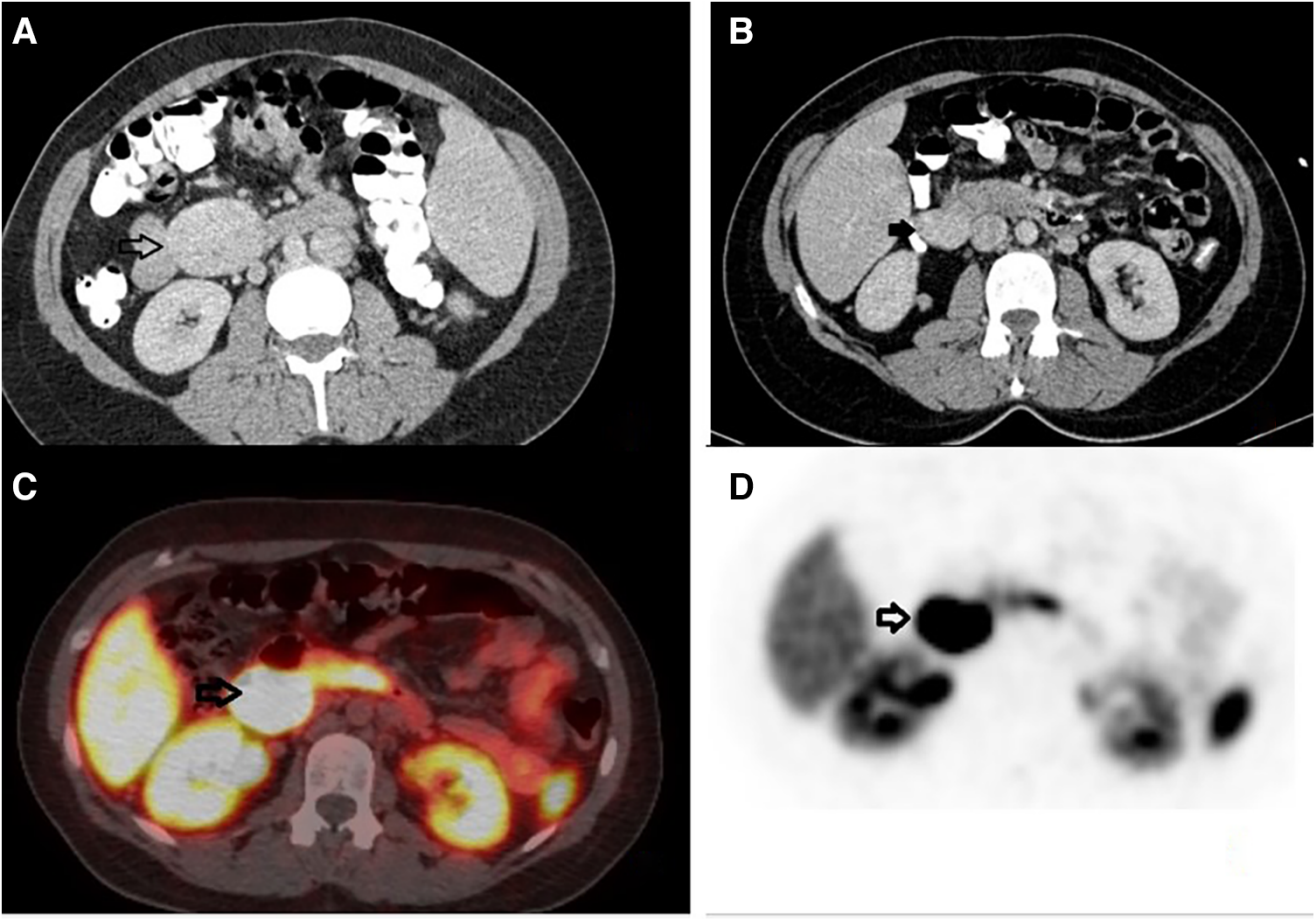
Preoperative imaging. (A) Computed tomography with intravenous contrast, transverse view, black arrow: an enhancing retroperitoneal mass, 4.1 cm in diameter, with clear boundaries, located in the left anterior pararenal space. (B) Computed tomography with intravenous contrast, transverse view, black arrow: an enhancing mass, 3.5 cm in diameter, located in the right retroperitoneal space. (C, D) Gallium-68 DOTATATE, transverse view, black arrows: a retroperitoneal mass, 3.5 cm in diameter, located medially to the upper pole of the right kidney, with increased uptake of the radioactive agent (SUV_max_ = 61), indicative of a neuroendocrine tumor.
